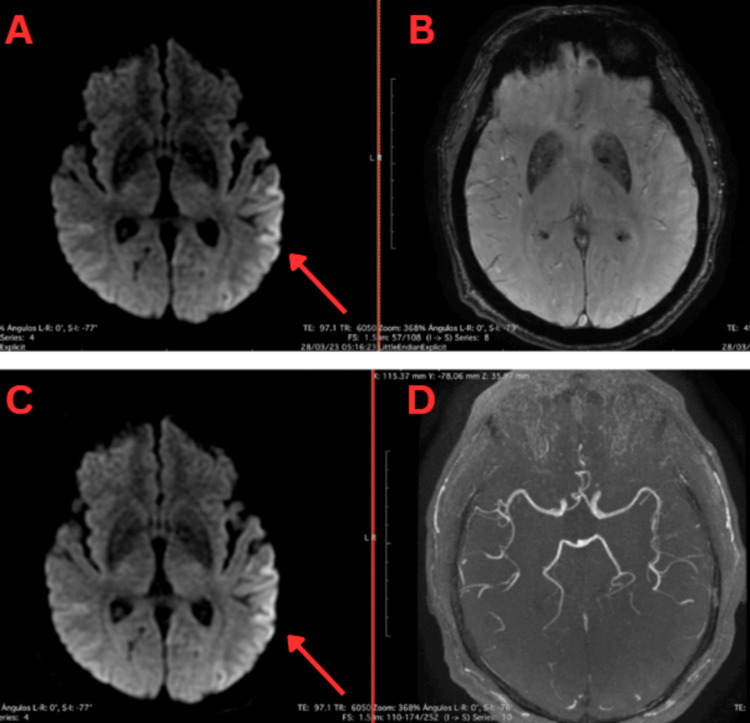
First axial brain MRI: diffusion-weighted, SWI, and MR angiography sequences Panels A and C (DWI): Diffusion sequence with apparent hyperintensity in the left posterior-parietotemporal cingulate gray matter and possibly the right temporal region, without contrast enhancement zones. Panel B (SWI) does not show significant hemorrhagic changes, and the absence of signal loss suggests no major hemorrhage. Panel D (MRA) shows intact vasculature.
